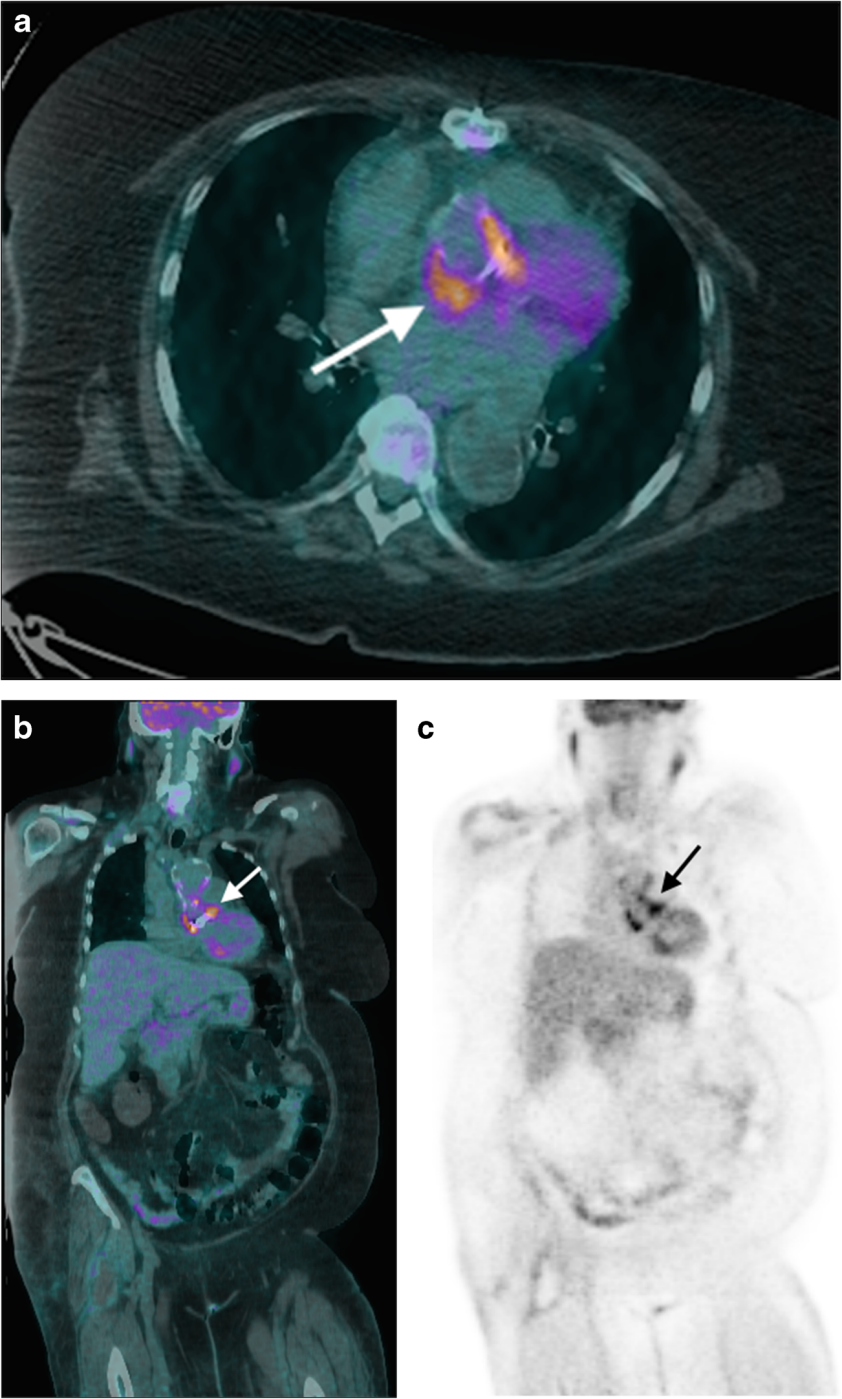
A 65-year-old woman presents with fever and dyspnoea 6 years post-mechanical aortic valve replacement and aortic root replacement for a type A aortic dissection. Blood cultures grew *S. aureus*, and the patient was referred for a TOE, which was equivocal. (a) Axial and (b) coronal fused 18 F-FDG PET/CT images and (c) coronal unfused 18 F-FDG PET images demonstrate intense FDG around the prosthetic aortic valve and at the aortic root (*arrow*) consistent with prosthetic valve endocarditis. The patient underwent reoperation for aortic valve replacement and aortic root repair
